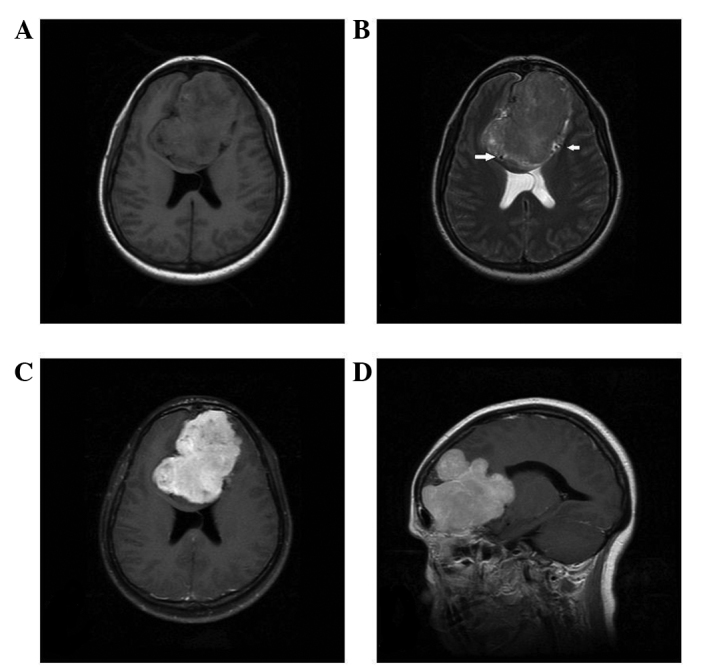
Patient 4. (A) Unenhanced magnetic resonance imaging scan reveals that the lesion is located in the anterior skull base with a well-defined border and lobulated shape. (B) The lesion exhibits a crow-midline growth pattern and intratumoral vessels are visible (arrow). (C) Enhanced scan reveals that the lesion is heterogeneously enhanced. (D) A narrow-based attachment to the skull base may be observed.
